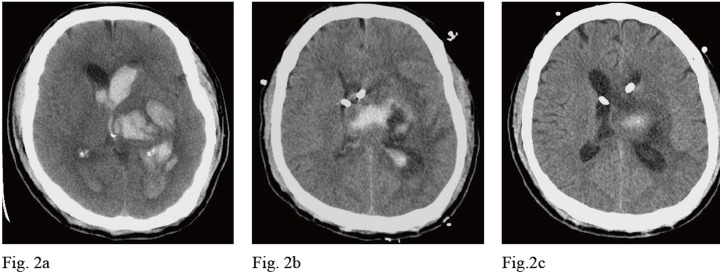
The change of brain CT scans in a spontaneous ICH patient (case 1) in 9 days. Fig 2a shows that the initial brain CT revealed left thalamic hemorrhage, intraventricular hemorrhage, and bilateral dilated ventricle. Fig 2b shows that on POD 6, the brain CT revealed that left thalamic hemorrhage was stationary. The brain parenchyma was tight, especially on the left side. Severe peri-hematoma edema was noted and the brain was shifted to right side. Two EVD tubes were placed in the bilateral frontal horns and the ventricles became slim. Fig 2c shows that the follow-up brain CT on POD 9 revealed that the hematoma and perifocal edema regressed with significantly less mass effect. The brain parenchyma became slack with clear margin of sulci. The EVD tubes were in bilateral enlarged lateral ventricles.
